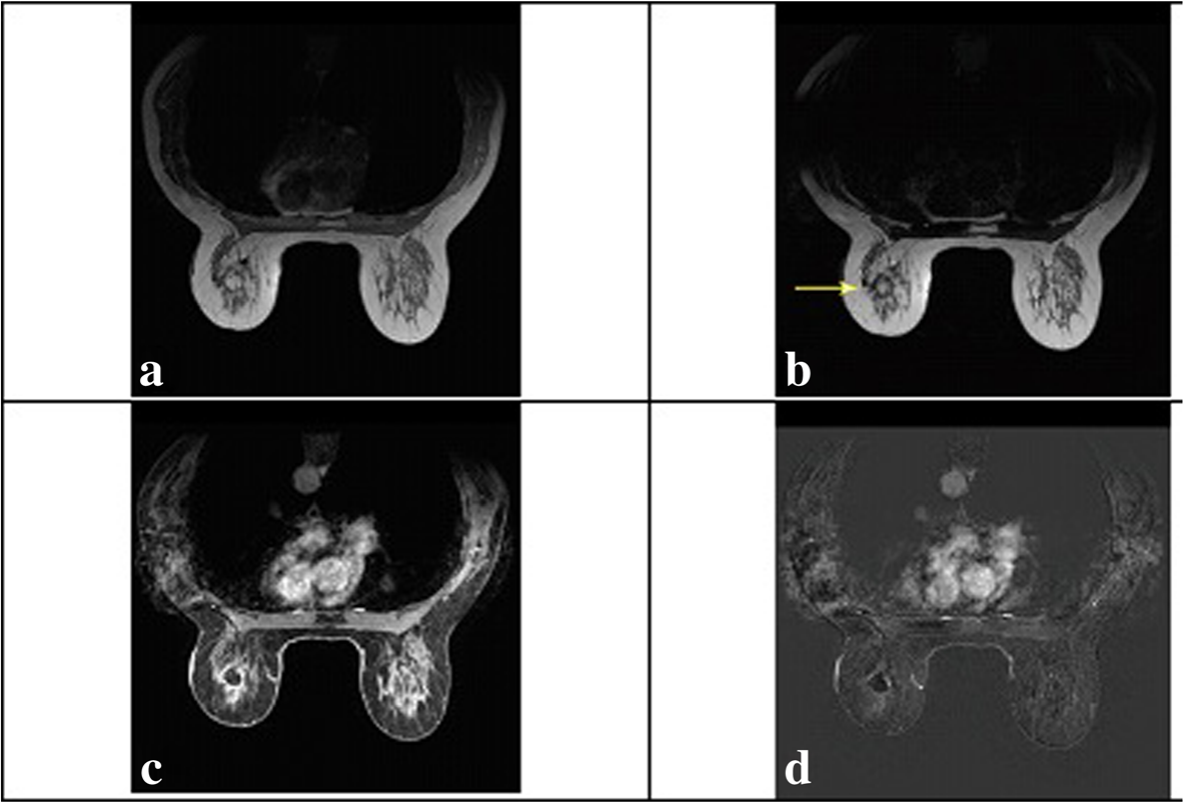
A patient who shows a mass in the left breast which follows fat signal on all sequences (arrow). a) Axial T1-weighted nonfat saturation, b) T2-weighted nonfat saturation, c) T1-weighted fat saturation after gadolinium and d) subtraction images (Derived in accordance with William et al. [19])
